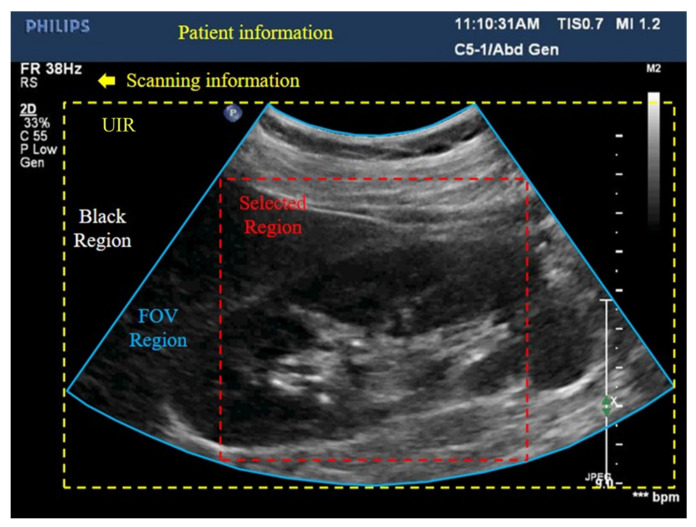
Digital scan-converted ultrasound image (kidney) shown on display. This ultrasound image (USI) region includes not only the field of view (FOV) region (blue box), but also the black region according to FOV and various information. To train ultrasound images, they are generally cropped to an USI region (yellow dotted rectangle) or selected region (red dotted rectangle).
